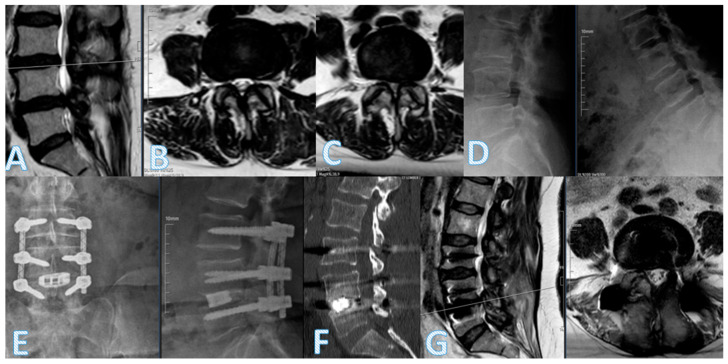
A 63-year-old female patient presented with severe low back pain (VAS 9) and left-dominant leg pain (VAS 8), accompanied by neurogenic claudication at 50 m. Preoperative sagittal T2-weighted MR (A), axial MR at L3–4 (B), and axial MR at L4–5 (C) demonstrate advanced disk degeneration and bilateral foraminal stenosis, most prominent at the L4–5 level. Dynamic flexion-extension lateral lumbar X-rays (D) show segmental instability. Postoperative anteroposterior and lateral radiographs (E), sagittal CT (F), and sagittal T2-weighted MR (G) confirm successful L4–5 transforaminal lumbar interbody fusion (TLIF) with a dynamic rod and interbody cage, showing restoration of disk height, decompression of neural structures, and stable implant positioning.
